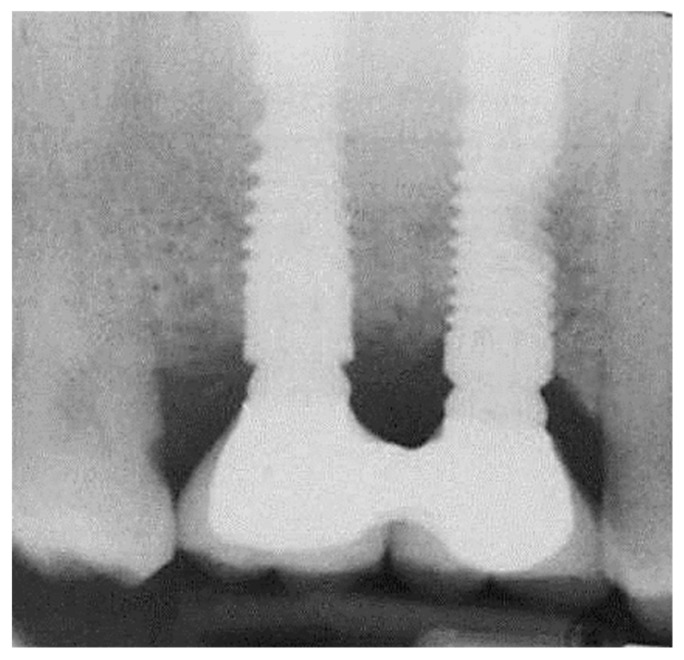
Periapical radiograph at the 3-year follow-up.
